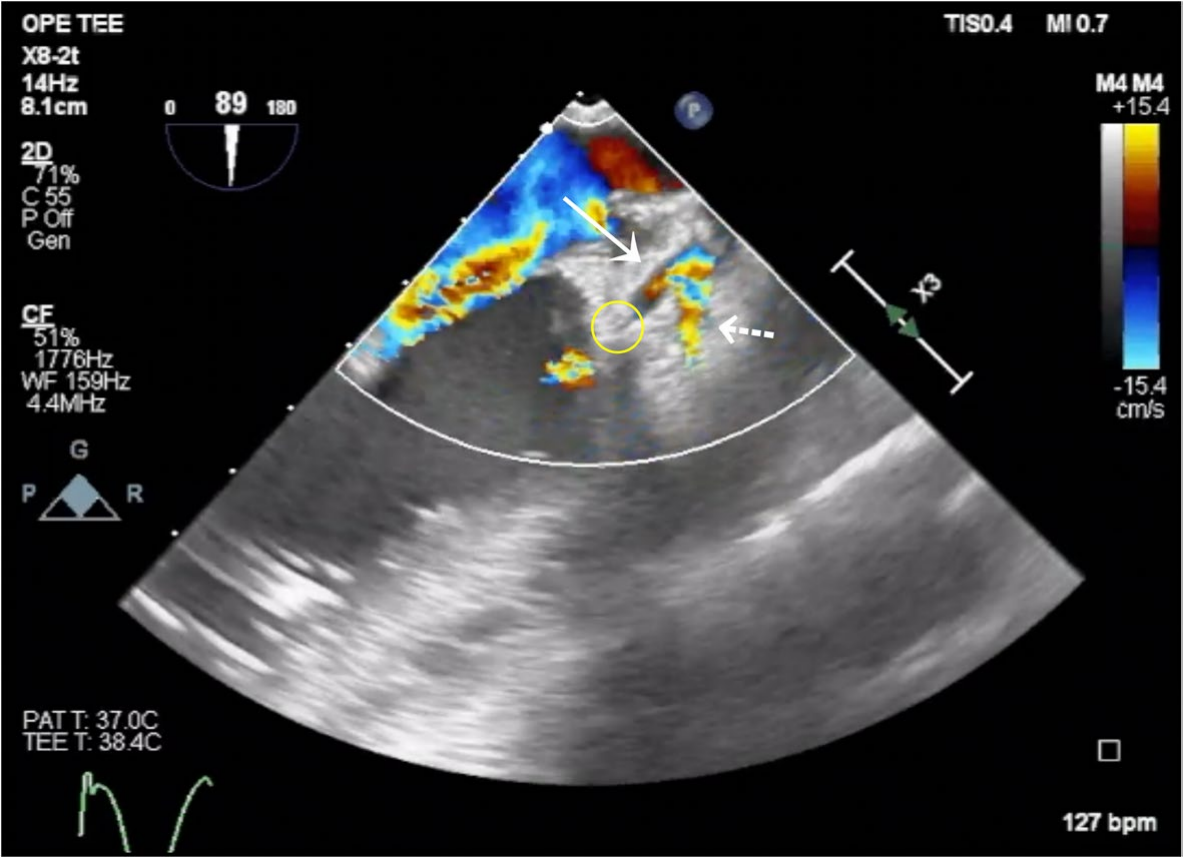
Flow interruption site in the left circumflex artery. Color Doppler image obtained using transesophageal echocardiography. The solid arrow indicates the left circumflex artery (LCx), and the dashed arrow indicates the left anterior descending artery (LAD). The yellow circle marks the site of flow interruption in the LCx
